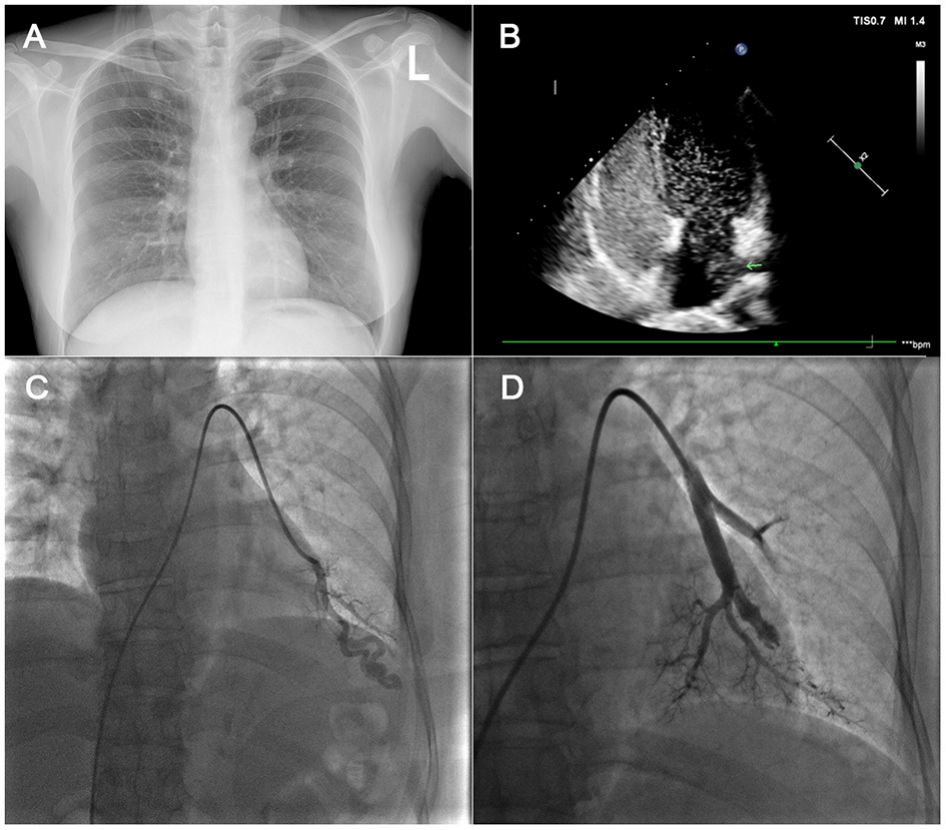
In (A): this patient had a chest X-ray with no abnormalities. In (B), the four-chamber image of CTTE showed markedly positive result and left chamber microbubbles came from the left lower pulmonary vein. In (C), a left lower lobe PAVM was noted on pre-treatment DSPA image. In (D), the PAVM was embolized. TTCE, transthoracic contrast echocardiography; DSPA, digital subtraction pulmonary angiography; PAVM, pulmonary arteriovenous malformation.
